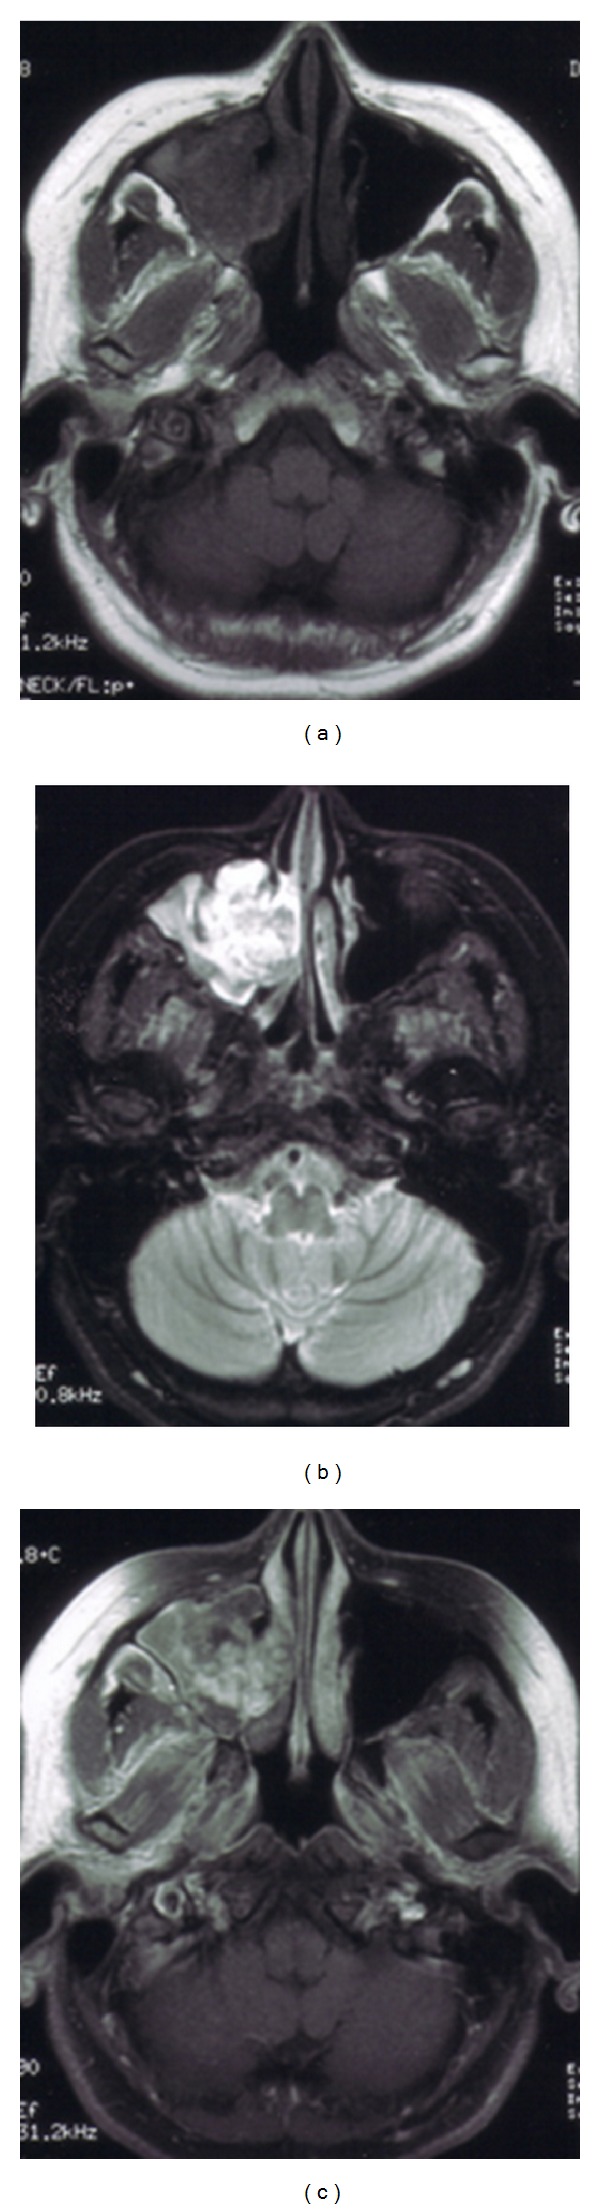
A 36-year-old female had a 2-month history of recurrent epistaxis. (a) Initial T1-weighted magnetic resonance image before treatment, showing a slightly low intensity in the central region of the mass (coronal view). (b) Initial T2-weighted magnetic resonance image before treatment, showing a heterogeneous mass. The central portion had high signal intensity, and the surrounding region had lower signal intensity than the central portion. The paranasal mucosa was thickened but kept its structure (axial view). (c) Initial Gd-DTPA magnetic resonance image before treatment, showing that the central portion was strongly enhanced. The surrounding region was less enhanced than the central portion. The thickened mucosa of the paranasal sinus was also strongly enhanced (axial view).
